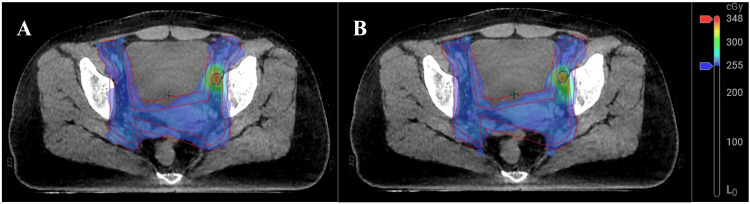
Comparison of the adapted plan and scheduled plan using iterative cone beam computed tomography-guided oART for this patient with LACC. Adapted plan (A) and scheduled plan (B) for fraction two of treatment. Abbreviations: LACC, locally advanced cervical cancer; oART, online adaptive radiation therapy
